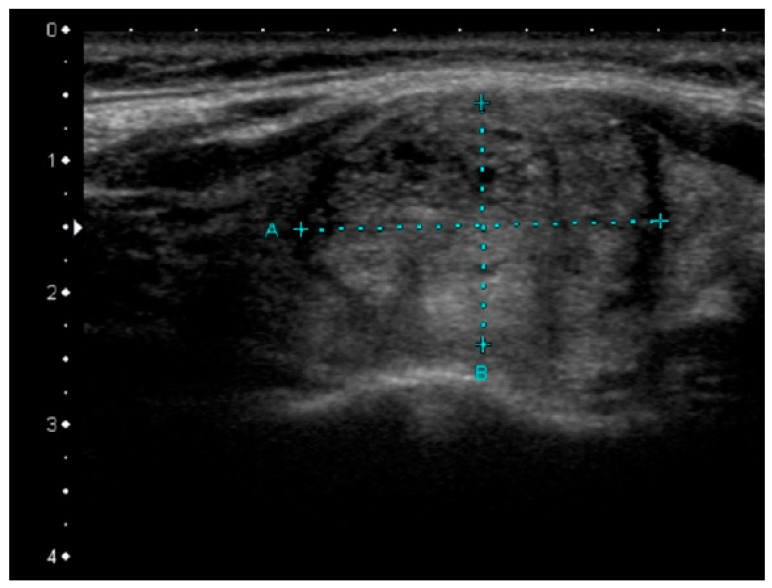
Sonographic pattern of *RAS*-positive papillary thyroid carcinima, slightly hypoechoic, heterogeneous lesion with rather well-defined margins.
